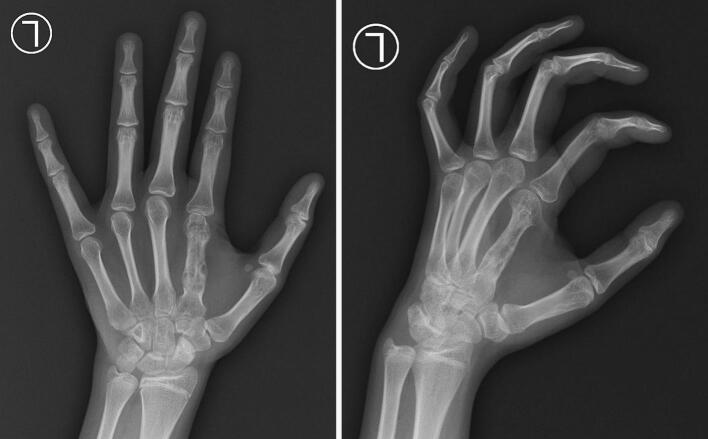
1-year follow-up, we conducted evaluation with X-ray, and there were no signs of recurrent GCT.
